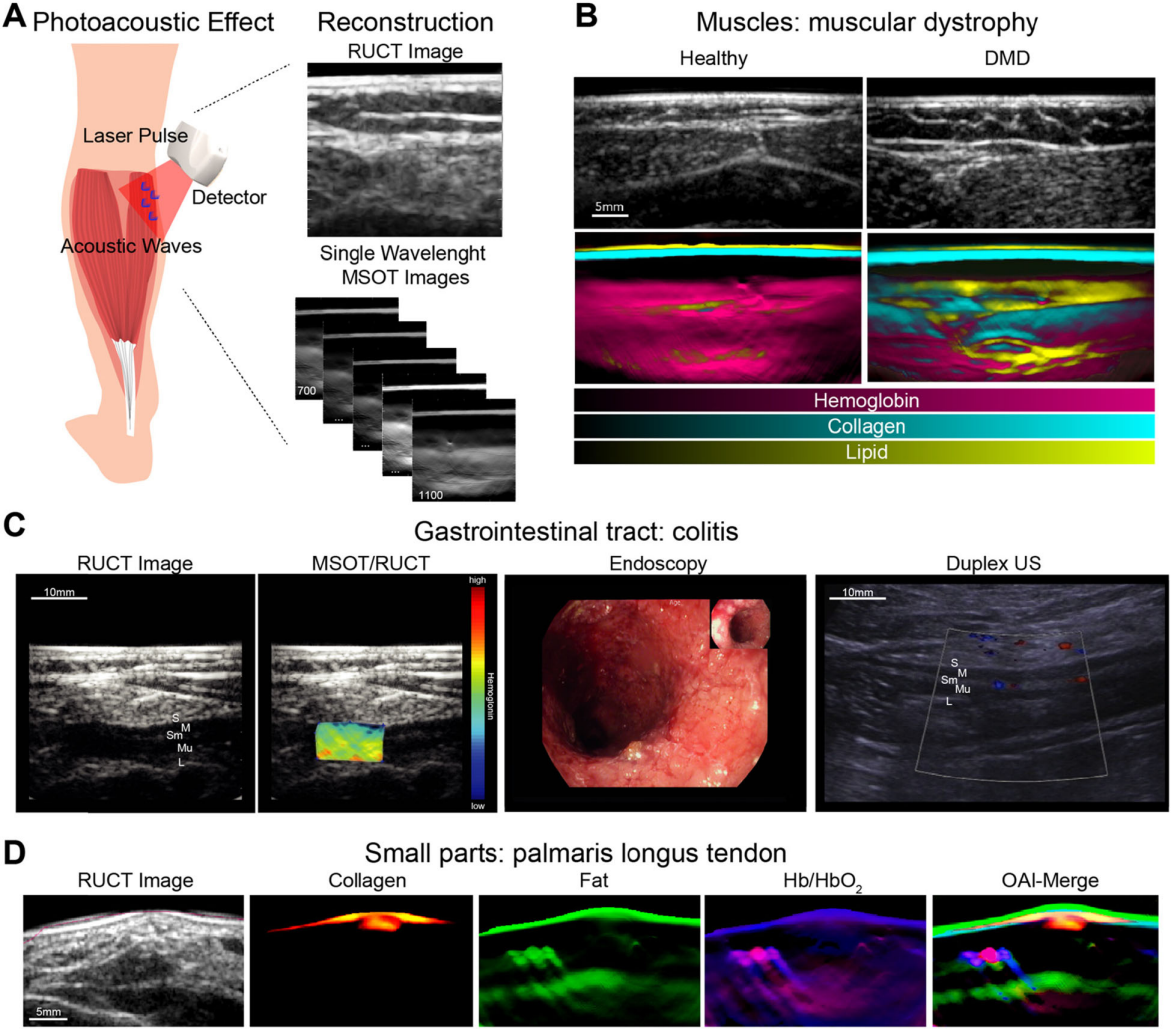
Principle of optoacoustic imaging. a The terms “photoacoustic” or “optoacoustic” describe the use of “light” and “sound.” The illumination of tissue by pulsed laser light and the subsequent recording of thermoelastic pressure waves combines the advantages optical (high contrast) and acoustic (high resolution) imaging. Subsequent image reconstruction and spectral unmixing enables non-invasive real-time visualization and quantification of specific endogenous chromophores such as hemoglobin, lipids, and collagens. RUCT reflectance ultrasound computed tomography, MSOT multispectral optoacoustic tomography. b Imaging example from muscular imaging resolving hemoglobin, collagen, and fat signals. DMD Duchenne muscular dystrophy. c MSOT imaging of the colon unmixed total hemoglobin signal. S serosa, M muscularis mucosa, Sm submucosa, L lumen, US ultrasound. d Example for imaging of palmaris longus tendon at the wrist with unmixing for different tissue components. Note the clear delineation of yellow/red containing tendon in comparison of hemoglobin signals from vessels
